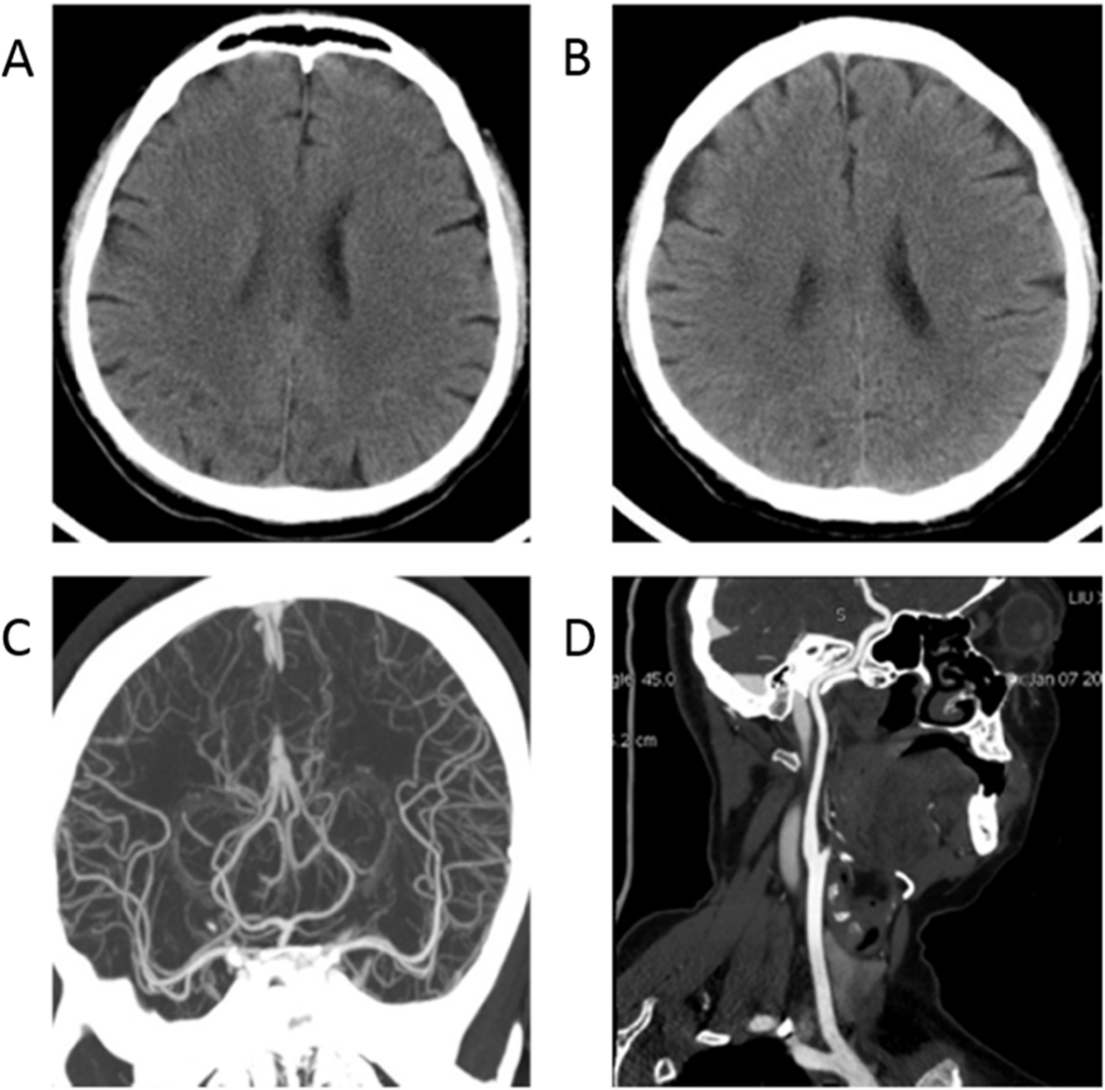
The head CT and head/neck arterial CTA of case 1 infected with SARS-CoV-2. a: Head CT on January 6, 2020, Illness day 8, b: Repeat head CT on January 8, 2020, Illness day 10; c: Head arterial CTA on January 6, 2020, Illness day 8, d: Neck arterial CTA on January 6, 2020, Illness day 8
